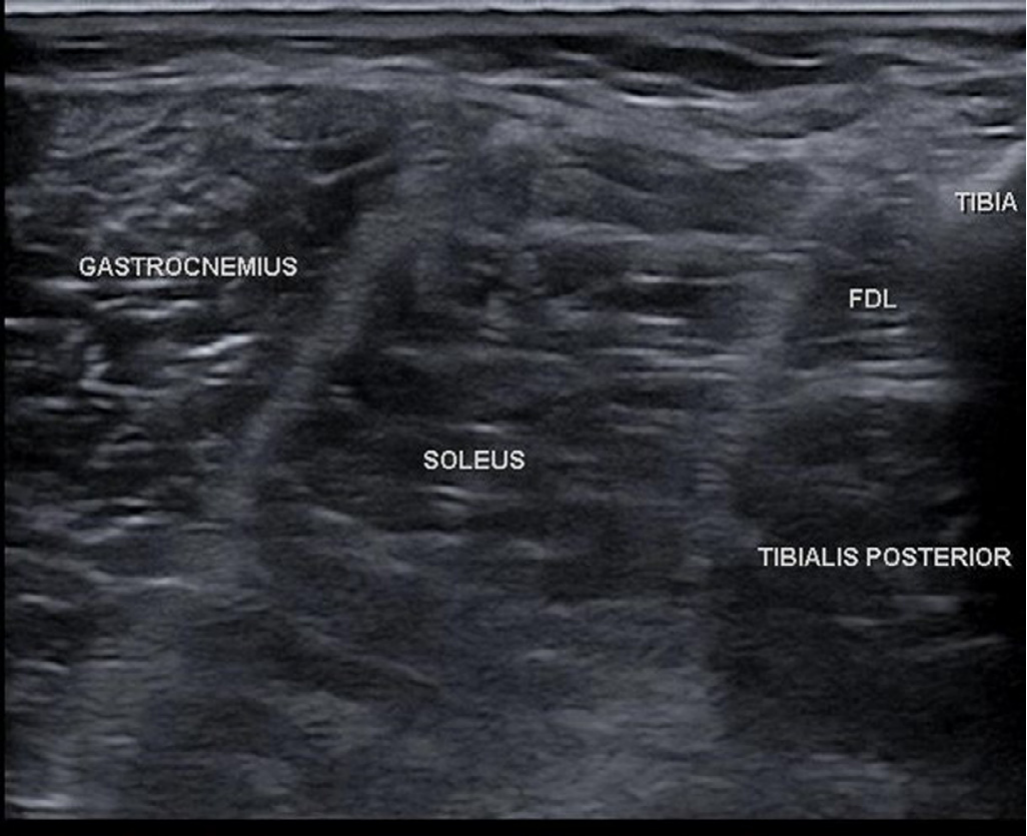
Ultrasound anatomy of the right flexor digitorum longus. The FDL is hypoechoic region deep to the soleus and superficial to the tibialis posterior.
